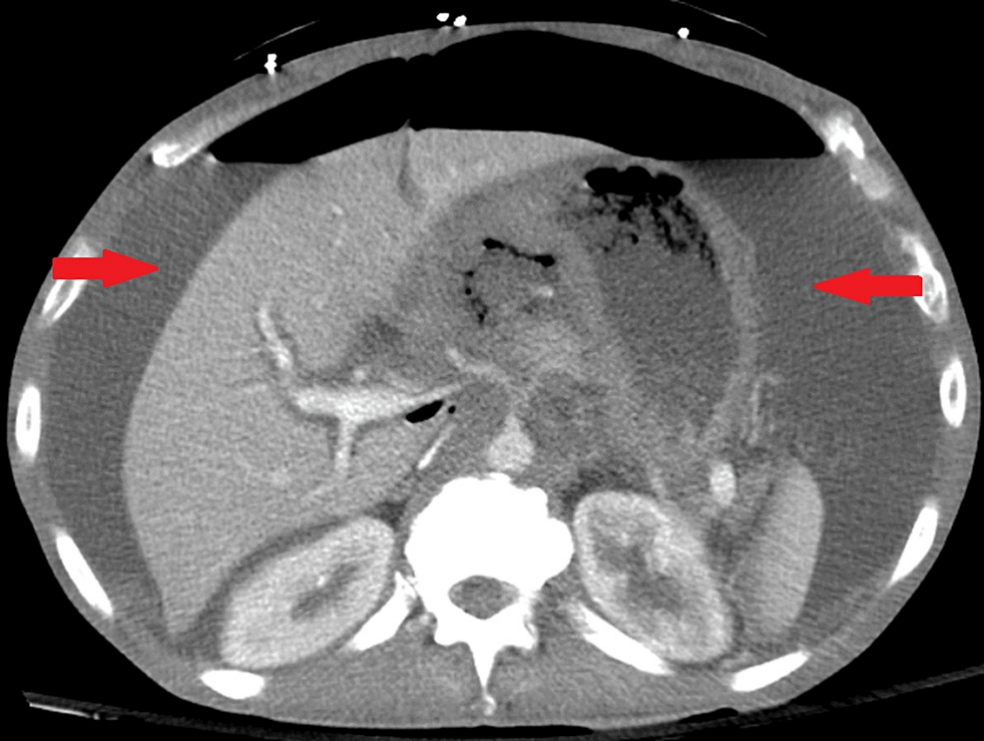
Computed tomography angiogram of the abdomen and pelvis showed large-volume pneumoperitoneum and ascites (arrow), suggestive of perforated viscus and peritonitis
